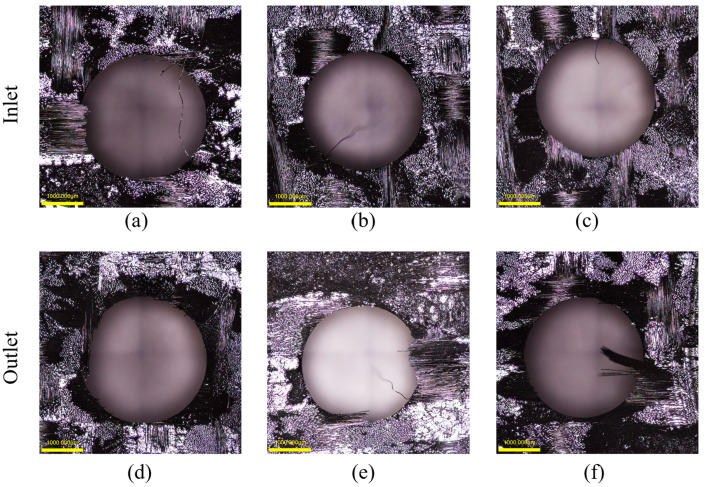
Inlet and outlet morphology of ultrasonic grinding holes at different feed rates. (a) F = 50 mm/min; (b) F = 200 mm/min; (c) F = 500 mm/min; (d) F = 50 mm/min; (e) F = 200 mm/min; (f) F = 500 mm/min.
